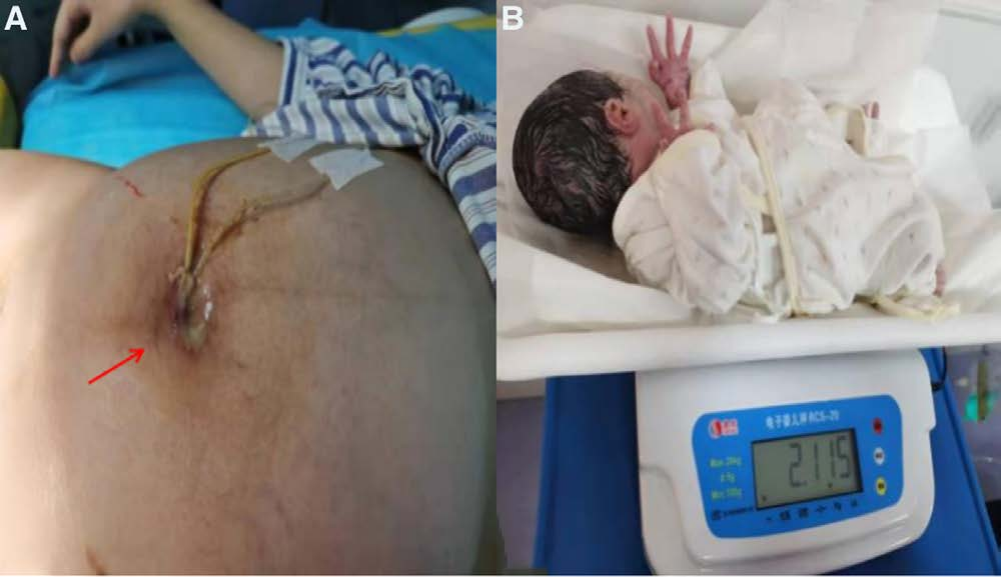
(A) Color Doppler ultrasound-guided puncture and drainage of periumbilical abscess. (B) Uterine water sac was used to induce labor, and a baby girl was delivered smoothly under the protection of perineum. The fetal position was LOA, Apgar score was 10, weight was 2115 g, length 45 cm, and appearance was not deformed. LOA = fetal position left anterior occipital.
